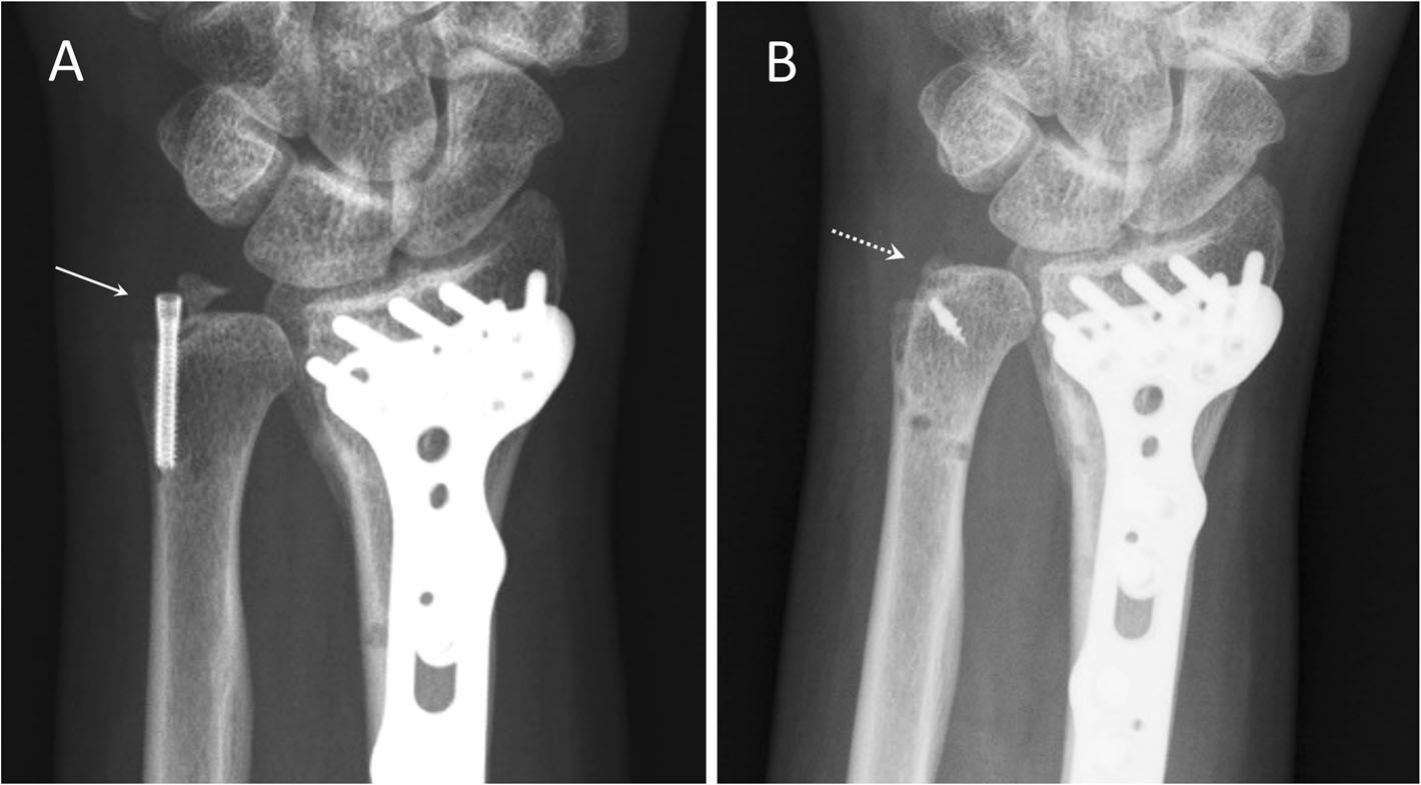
Posteroanterior radiographs of a 35-year-old male patient receiving open reduction and internal fixation for distal radioulnar fracture. a Ulnar styloid displacement with nonunion (arrow) at 4 months after screw fixation. b Osseous union (dotted arrow) at 3 months after revision fixation with anchor suture fixation
